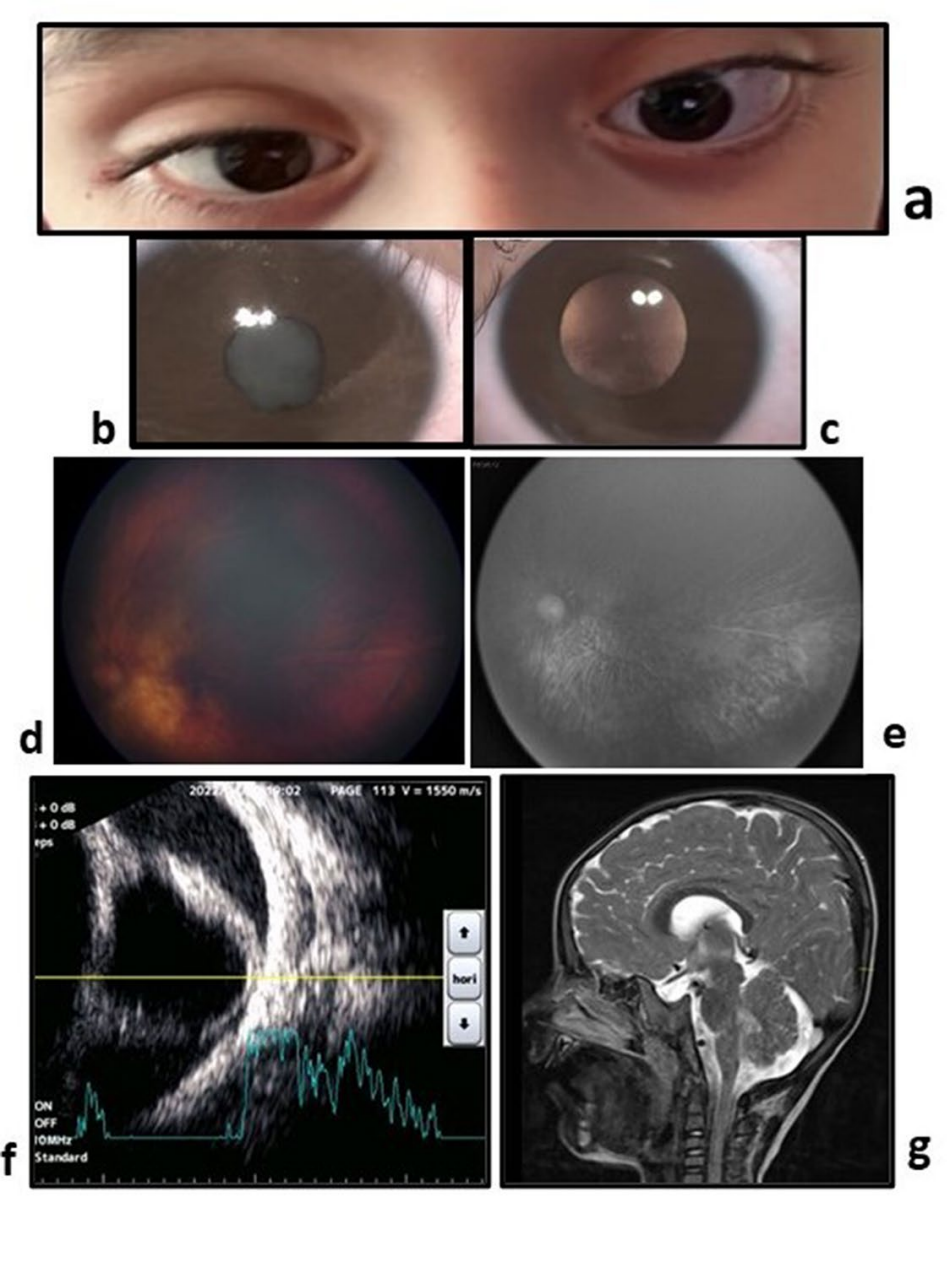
a- Esotropia b- Anterior segment photograph of the right eye, posterior synechia, and cataract c- The left eye, with mid-dilated pupil and clear lens d- fundus photo of the left eye and tessellated appearance associated with high myopia e- Lobular filling and attenuated vessels in FA f- Retinal detachment and thickening on B-scan USG g- Cranial Sagittal T1-weighted (T1W)MRI: Occipital fat pad and midline occipital defect
